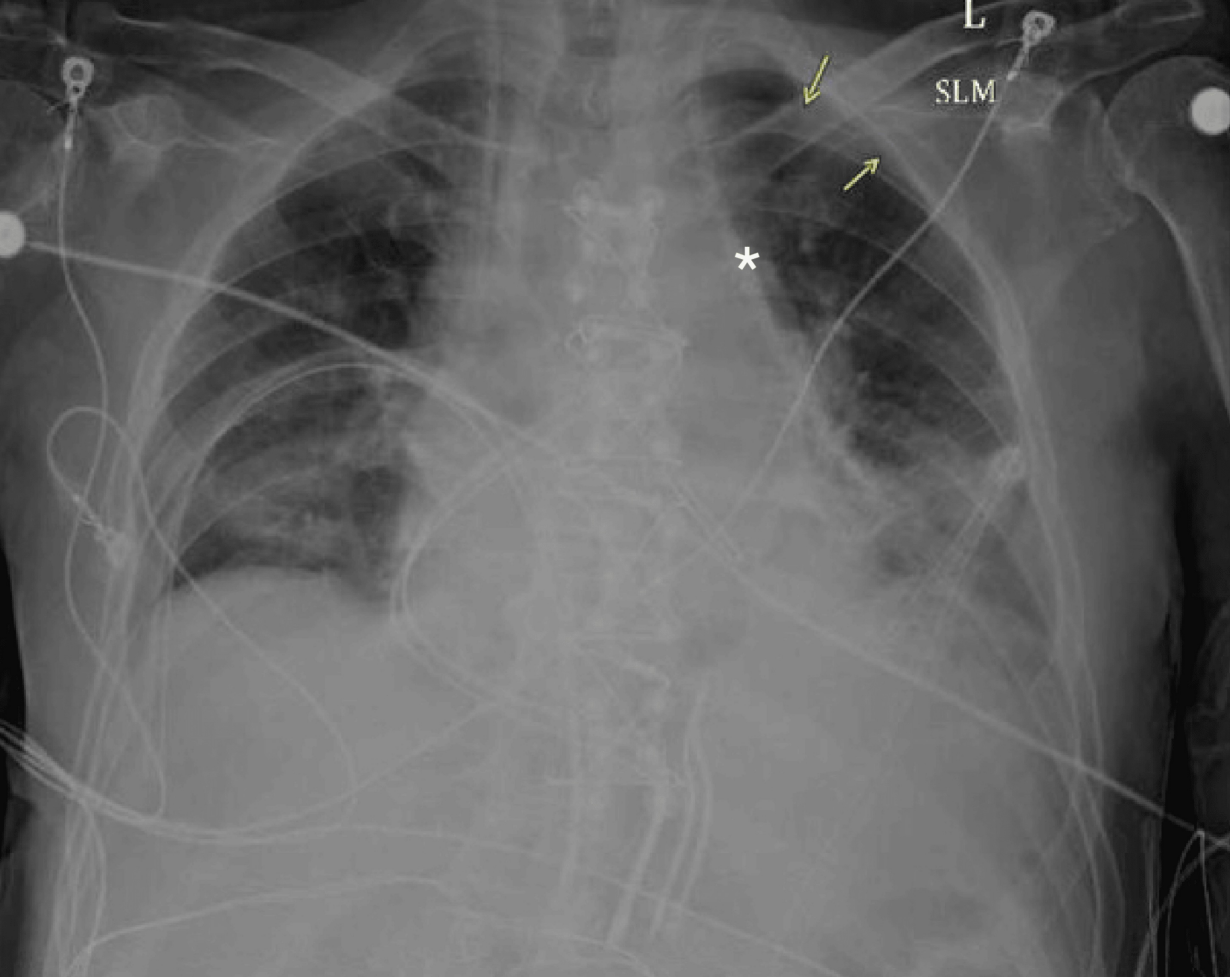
Plain chest x-ray showing malposition of left internal jugular central venous catheter placement Central venous catheter seen extending to left mediastinum (star). Small pneumothorax also present unchanged from before line placement (yellow arrows).
